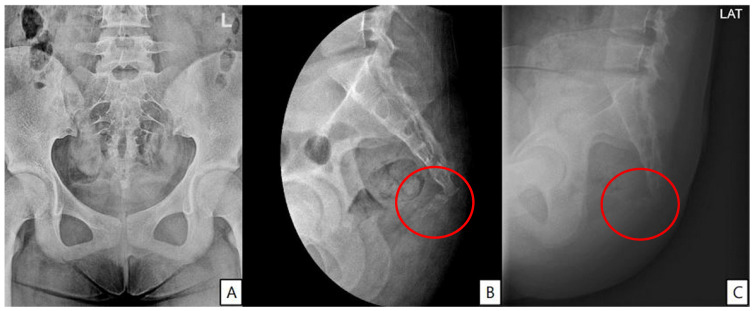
(A) Anteroposterior pelvic radiograph showing no visible fracture but overall coccygeal axis deviation. (B) Dynamic lateral view showing anterior angulation of the coccyx at the sacrococcygeal junction (red circle). (C) Seated lateral view demonstrating accentuated anterior displacement, suggestive of coccygeal instability (red circle).
